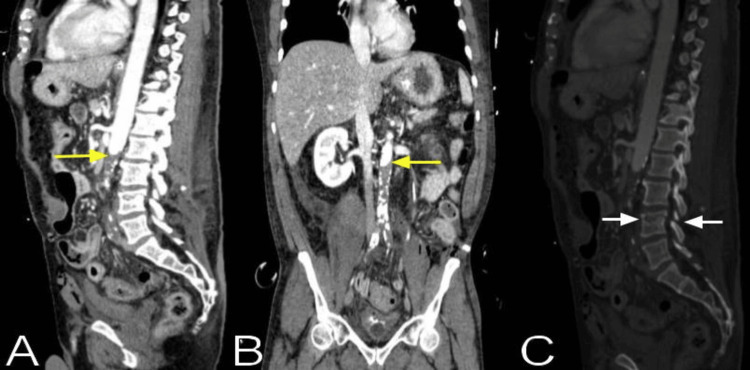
The abdominal CT angiography sagittal (A) and coronal (B) views show an abrupt cutoff of the infrarenal aorta (yellow arrows) with an occluding thrombus immediately below the take-off of the left renal artery at the level of the L2-L3 vertebral junction without distal reconstitution, with significant aortic atherosclerosis changes below the occlusion. The CT bone reconstruction, sagittal (C) view, demonstrates an L4 superior vertebral body fracture, with the fracture line extending throughout the superior endplates and bilateral pedicles (white arrows).
